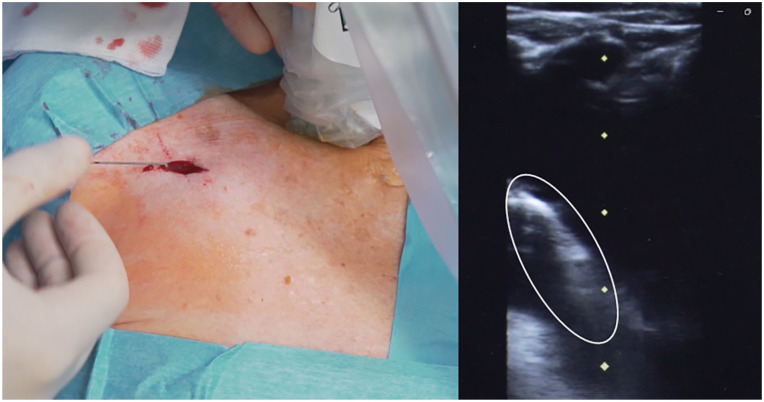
Ultrasound-based tip navigation: scan of the veins of the supraclavicular area according to the ECHOTIP protocol.
